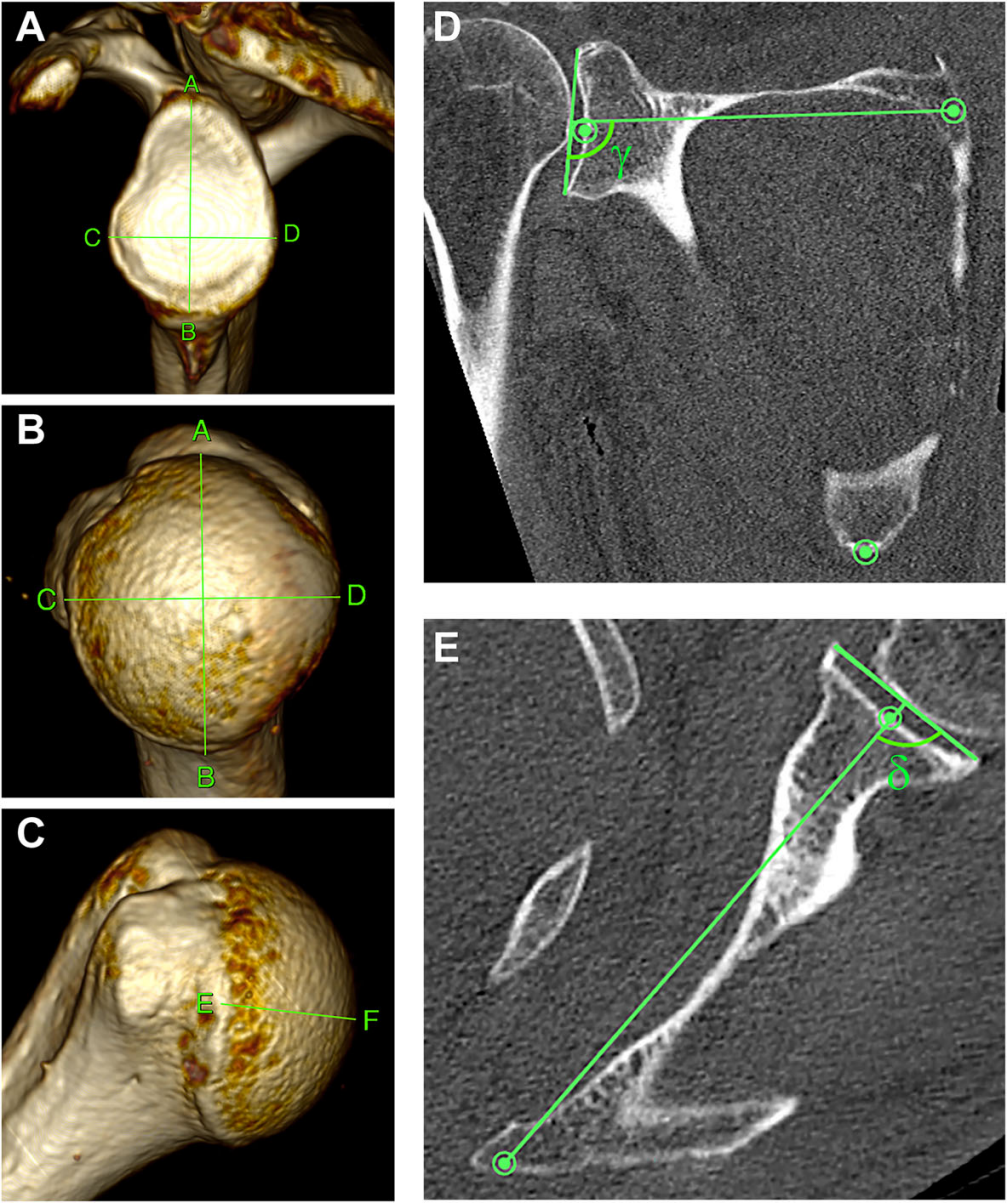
2D-CT images of 3D-CT reconstructions of the articular surfaces of a left shoulder. A Height and width of the glenoid measured as described [35]: a line drawn from the most cranial (A) to the most caudal point (B) of the glenoid cavity, and another line from the most ventral (C) to the most dorsal point (D) of the glenoid rim. B Height and width of the humeral head measured using established landmarks [26]: the greater tubercle as reference to place the uppermost point (A) at the edge of the smooth articular surface and a perpendicular line was drawn to the lowest (B) point. C The depth of the humeral head measured using established landmarks [26]: the lesser tubercle as reference for the most anterior point (F), from this, a perpendicular line was drawn to the most posterior point (E) of the smooth articular surface. D The inclination angle α was determined in the coronal plane using reference points (green) as described [7, 35]: a line was drawn through the root of the scapular spine to the midpoint of the glenoid cavity, and a second line through the most cranial and caudal points (see A, reference points A and B) of the glenoid rim. Angle γ was measured between the axes of these two lines in caudal direction, and the inclination angle α calculated: α = γ - 90°. E The ante−/retroversion angles β were determined in the transversal plane using reference points (green) as described [13, 35]: a line was drawn through the root of the scapular spine to the midpoint of the glenoid cavity, and a second line through the most posterior and anterior points (see A, reference points C and D) of the glenoid rim. Angle δ was measured between the axes of these two lines in sagittal direction and angle β calculated: β = δ - 90°
